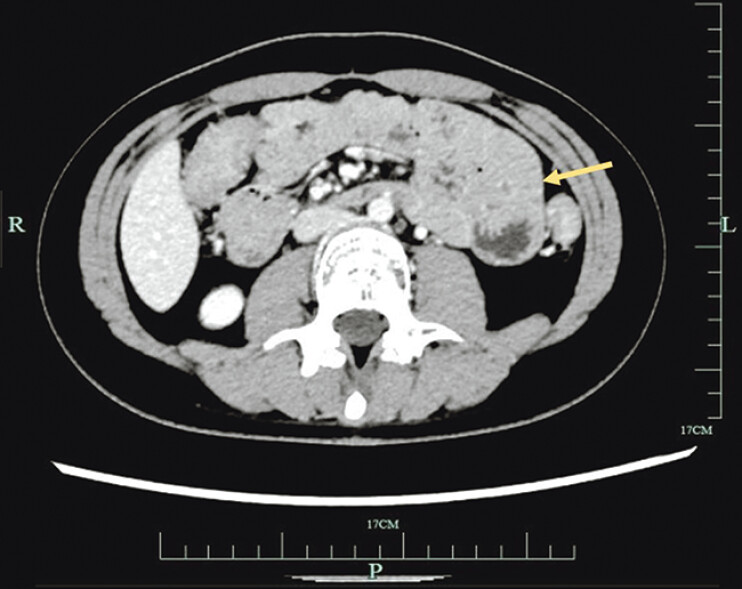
Contrast-enhanced abdominal computed tomography revealing an intussusception-like appearance (arrow) of the duodenojejunal junction.
